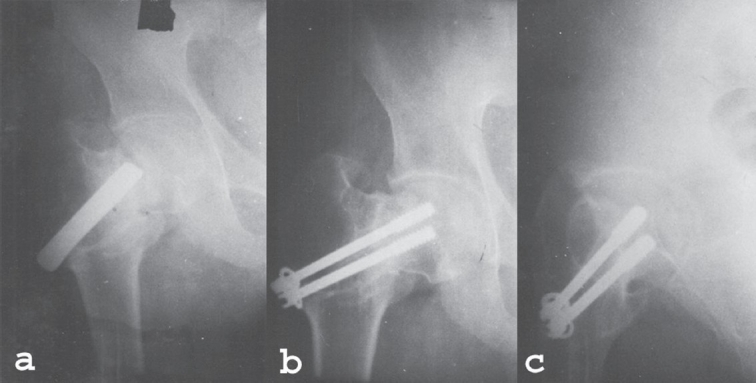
a,b,c: X-ray anteroposterior view showing internal fixation with non-union in a followup case (a), Three years followup X-ray showing sound union, implant *in situ,* coxa vara (b) and X-ray lateral view showing bony union (c)
